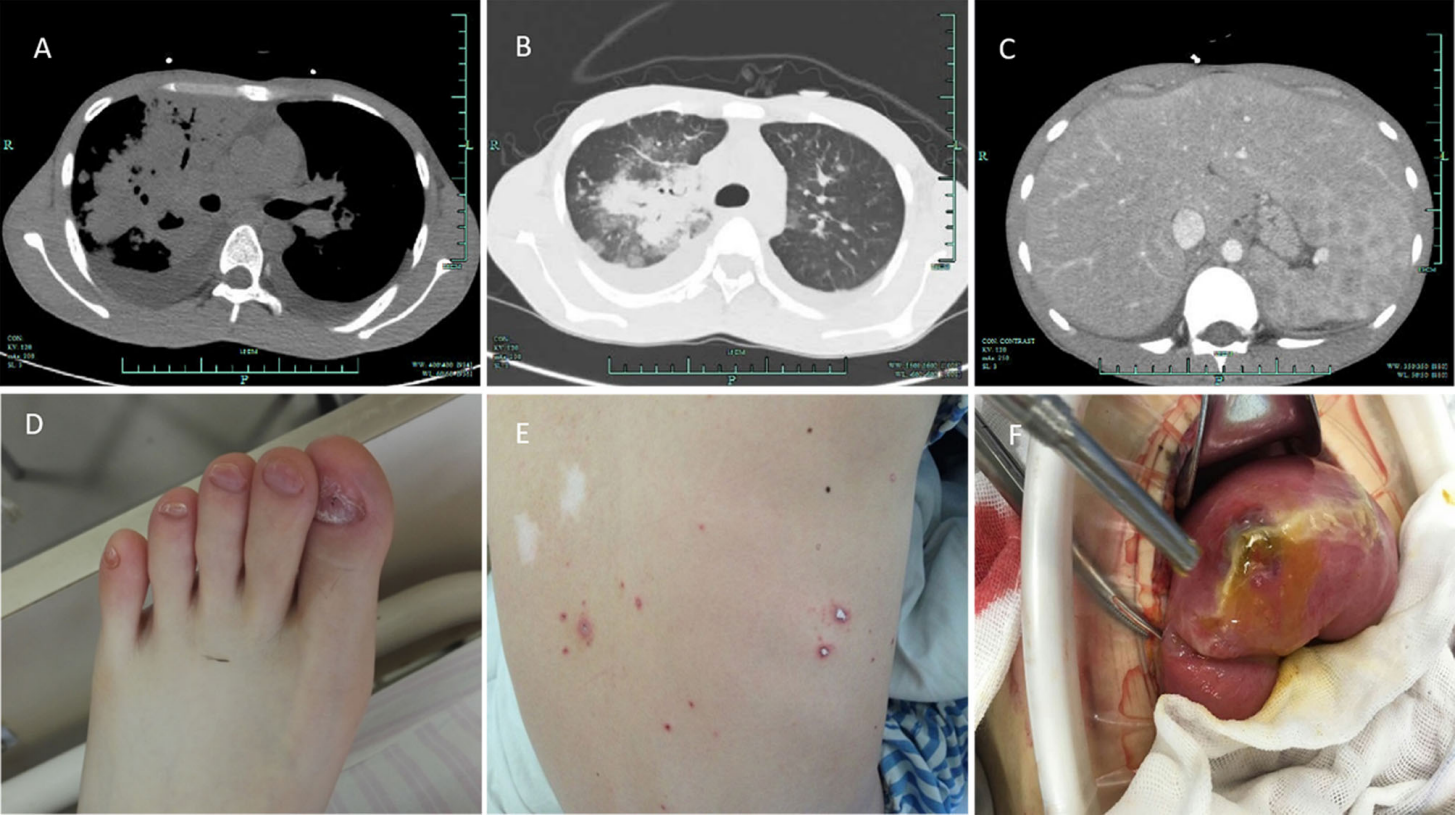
Clinical photos. (A) Bilateral lung infection with a bilateral pleural effusion, a paraspinal mass of the right lower mediastinum, and pericardial effusion. (B) CT of pulmonary Talaromyces marneffei infection. (C) Enlarged spleen, multiple splenic lesions, cholecystitis, and peritoneal effusion. (D) Abnormality of the patient's toenails. (E) Rash on the patient's back due to Talaromyces marneffei infection. (F) Surgical exploration demonstrated intestinal perforation and edema of the surrounding mucosa.
